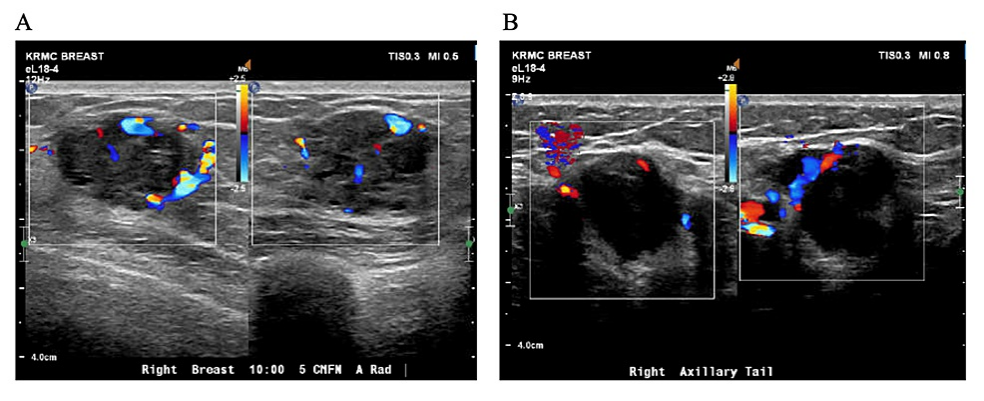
Right breast ultrasound. A right breast mass at 10:00, 5 cm from the nipple, measuring 2.4 cm (A) and a right axillary tail abnormal lymph node measuring 2.3 cm (B).
